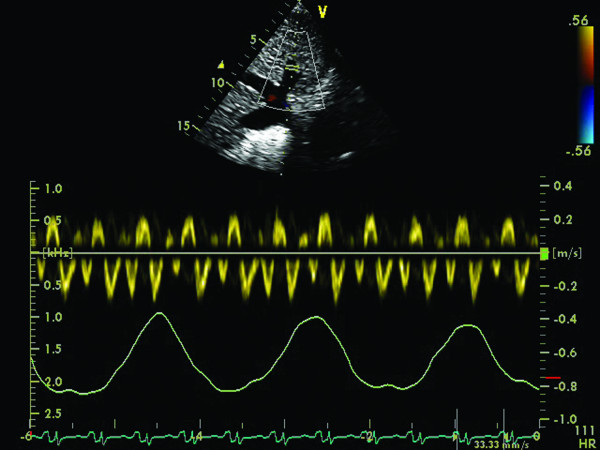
Constrictive pericarditis. Respiratory variation of Doppler curve of hepatic vein flow (minimal increase in S and D peak velocities during inspiration, increased A wave during expiration).
